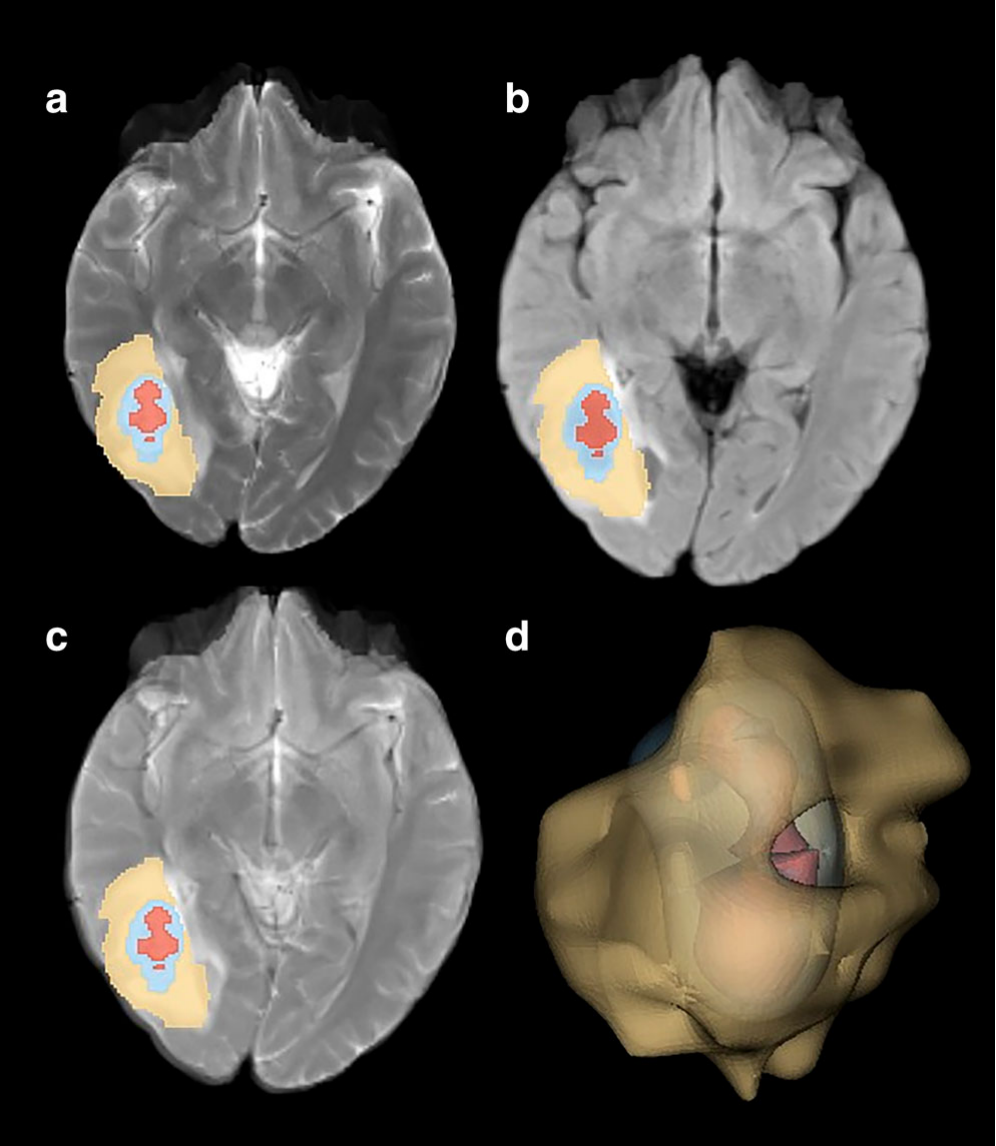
Brain metastasis from NSCLC located in the right cerebral hemisphere. (a) T2‐weighted images, (b) FLAIR‐weighted images, (c) T2‐FLAIR digital subtraction images, (d) 3‐dimensional volume rendering of the mass yellow color: perilesional edema area, blue color: contrast‐enhanced area, red: necrosis area.
